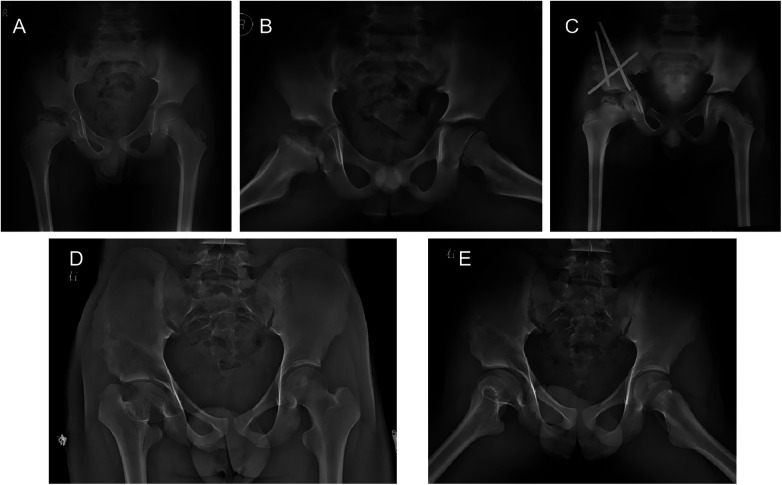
Serial radiographs of a 7-year-10-month-old boy with right femoral head necrosis in the early reossification stage treated with containment surgery. (A) Initial anteroposterior pelvic radiograph showing the lesion before treatment; the affected hip was the right hip. (B) Preoperative frog-leg lateral radiograph demonstrating loss of containment of the femoral head on the right side. (C) Early postoperative radiograph of the right hip after containment surgery. (D) Follow-up radiograph showing continued healing and remodeling of the right hip. (E) Radiograph at the final follow-up of 6 years and 9 months, showing the outcome of the right hip, which was classified as Stulberg grade III (class II).
